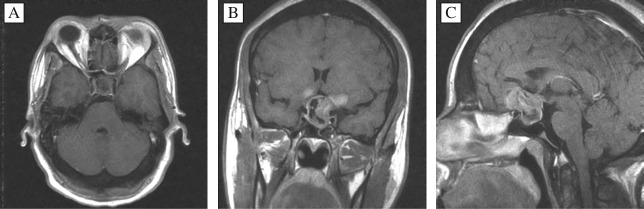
Preoperative T1-weighted axial (A), coronal (B) and sagittal (C) gadolinium-enhanced MRI scan.
